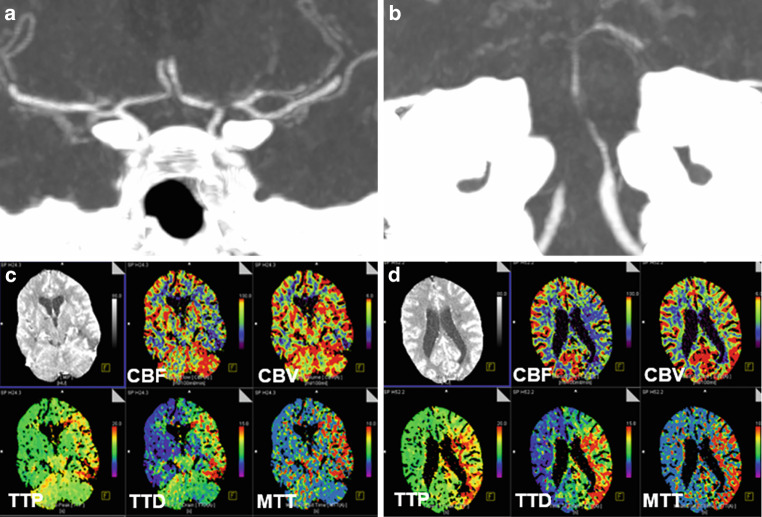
CTA and CTP obtained on day 14 after the onset of right hemiplegia and altered consciousness of a patient with LNB-associated severe cerebral vasculitis. CTA images (coronal views; a, b) showing critical stenosis of the left ACA/A1, left MCA/M1/superior trunk, bilateral vertebral artery/V4, and the BA. The time-to-drain (TTD) and mean transit time (MTT), determined by CTP (c, d), is prolonged in the entire posterior circulation and the left anterior circulation with concomitant cerebral blood volume (CBV)-cerebral blood flow (CBF) mismatch
